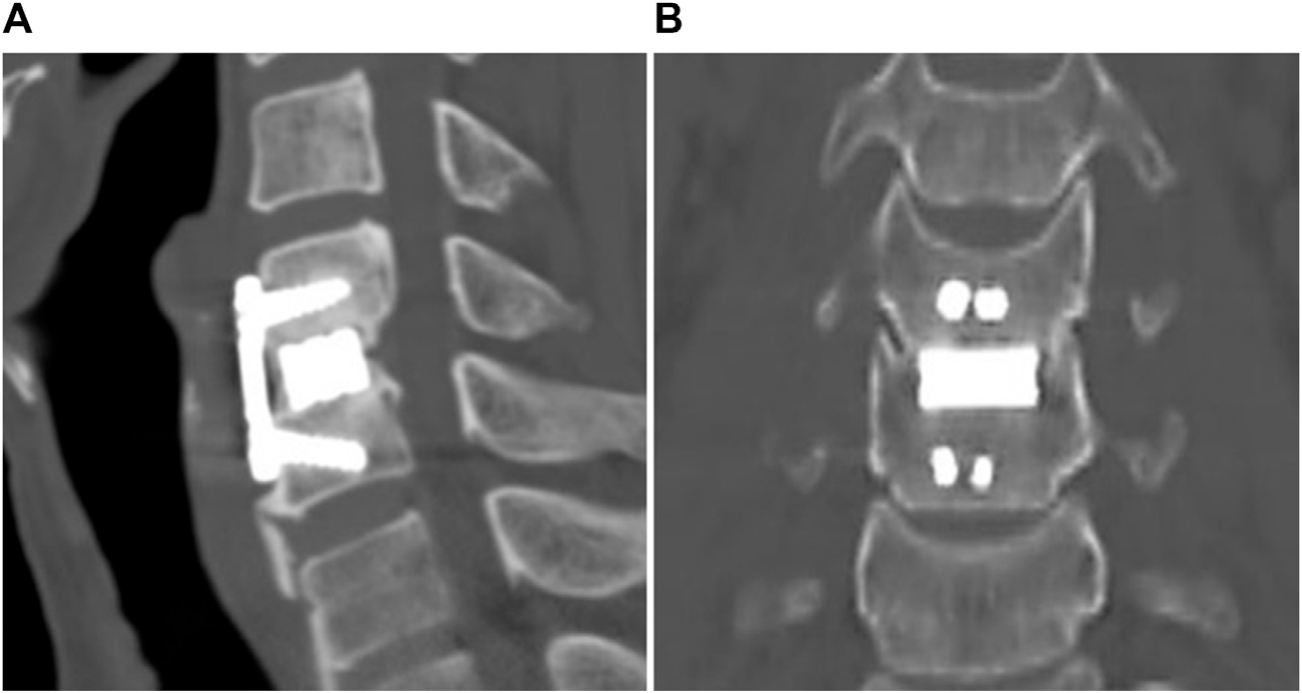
One year post-operative images from CT scan. (A) Sagittal view, (B) coronal view.
